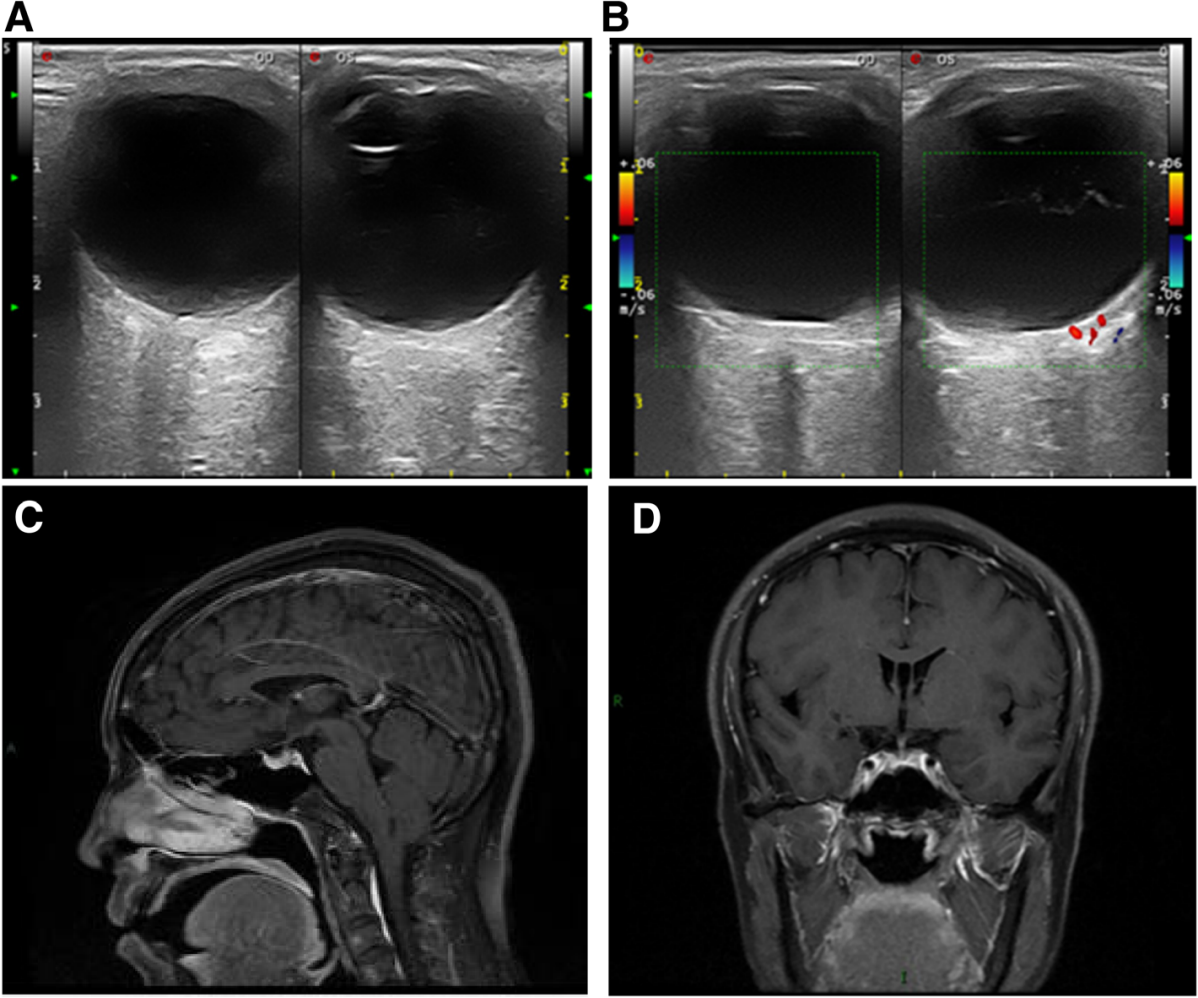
a B scan of two eyes revealed normal scleral thickness. No occupied lesions were indicated on sonogram. b Orbital color Doppler imaging showed no signs of abnormal blood flow, nor any reversal of flow. The head enhanced axial MRI image (c) and coronal scanning image (d), revealed no blood transfer between the cavernous sinus and intracranial artery in our case. No vascular malformation or space occupying lesion was observed
